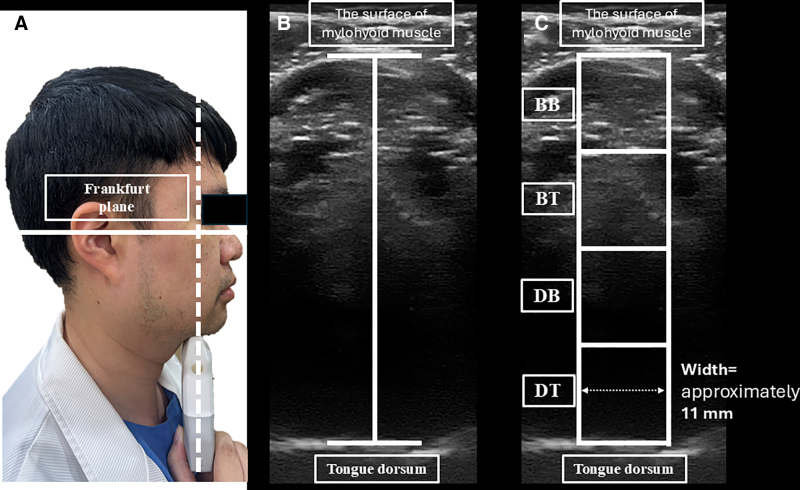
Ultrasonographic assessment of tongue thickness and echo intensity. (A) Participant positioning during tongue ultrasonography, with the head adjusted to maintain the Frankfurt plane parallel to the floor and the probe placed at the midline between the bilateral second premolars. (B) Measurement of tongue thickness on a midsagittal ultrasound image, defined as the vertical distance from the surface of the mylohyoid muscle to the dorsal surface of the tongue. (C) Definition of the ROI for echo intensity analysis, aligned with the axis of tongue thickness measurement. The ROI was vertically subdivided into 4 anatomical regions: top of the tongue dorsal side, bottom of the tongue dorsal side, top of the basal tongue side, and bottom of the basal tongue side. Only the BT region was included in the present analysis. The ROI width was standardized in image units and is shown here with an approximate millimeter scale for reference. BB = basal bottom, BT = basal tongue, DB = dorsal bottom, DT = dorsal tongue, ROI = region of interest.
